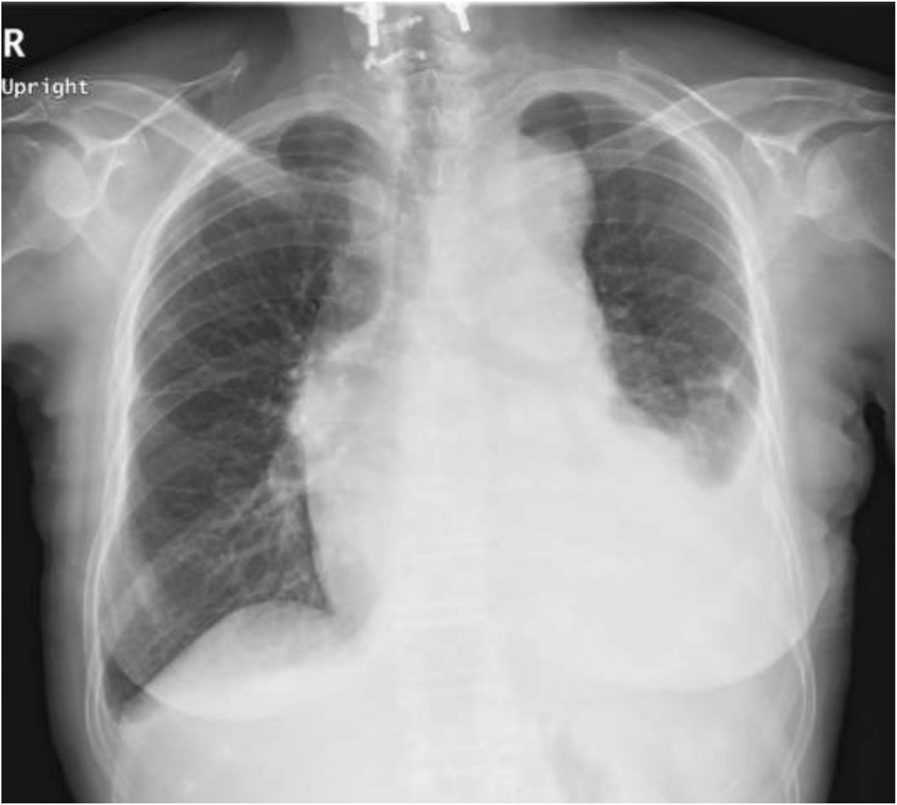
Preoperative chest X-ray showing blunting of the left costophrenic angle
